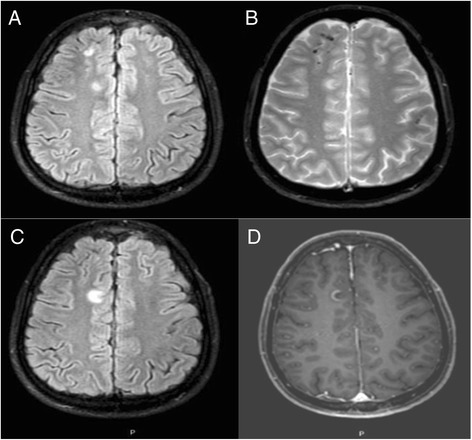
MRI images of 26 year-old patient with CAPS during a relapse with severe headaches. (a): Axial Flair images showing T2 FLAIR hypersignal right frontal juxta-cortical lesions with (b) hyposignal lesions on T2* weighted sequences suggesting hemosiderin deposits and (c) right anterior cingular gyrus T2 FLAIR hypersignal with (d) gadolinium enhancement
